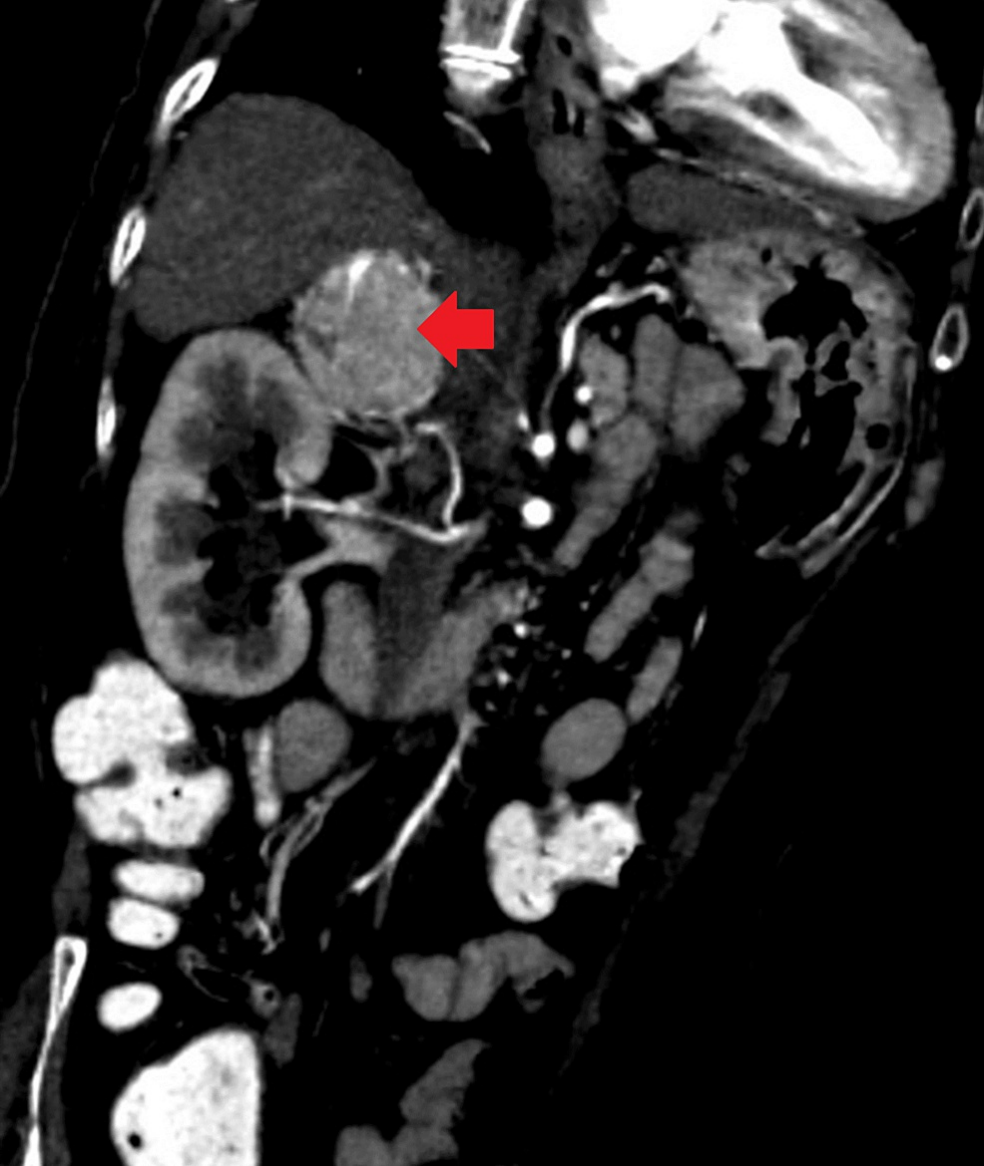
CECT of the abdomen showing well-defined iso-hypodense suprarenal lesion of size 45 mm x 40 mm x 35 mm arising from right adrenal gland marked by a red arrow. A well-defined iso-hypodense lesion arising from the right adrenal, located superior and anterior to the upper pole of the right kidney. The lesion is encased by the right suprarenal artery and located posterior to the inferior vena cava, compressing and displacing it anteriorly. CECT: contrast-enhanced computed tomography; mm: millimeter
